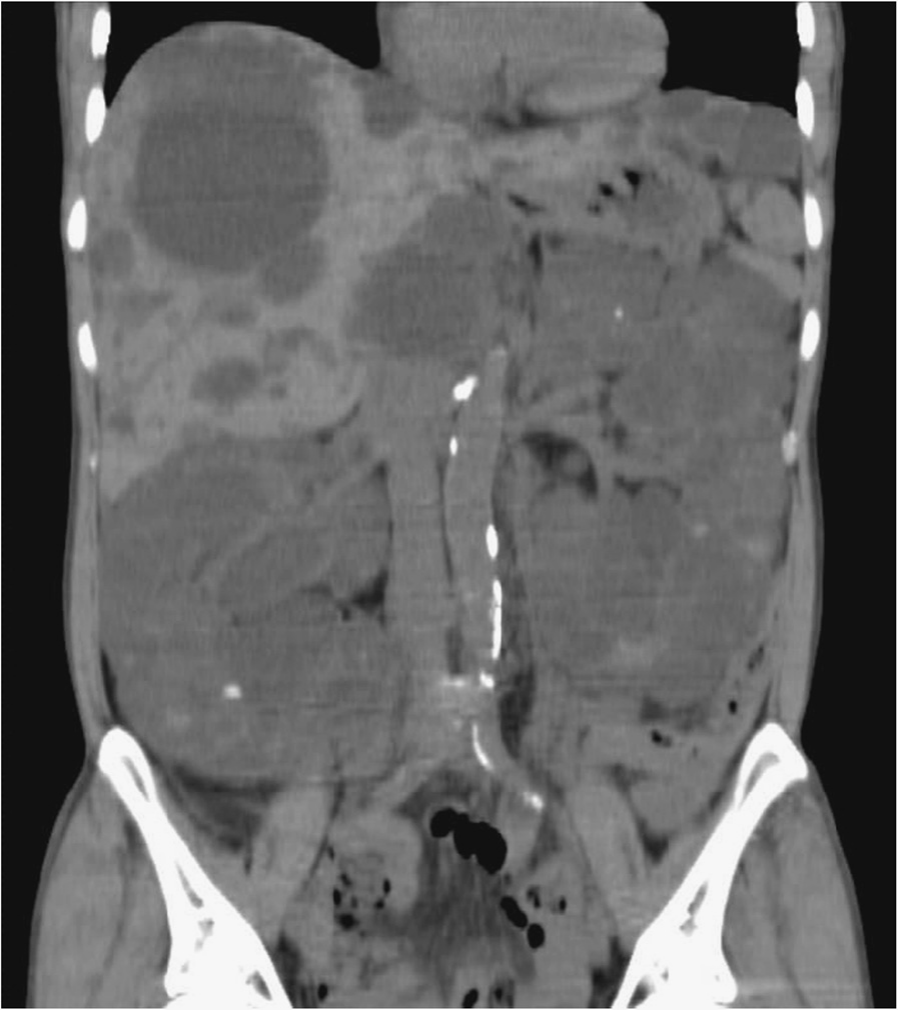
Abdominal computed tomography shows an enlarged liver and kidneys with multiple cysts in case 1.
